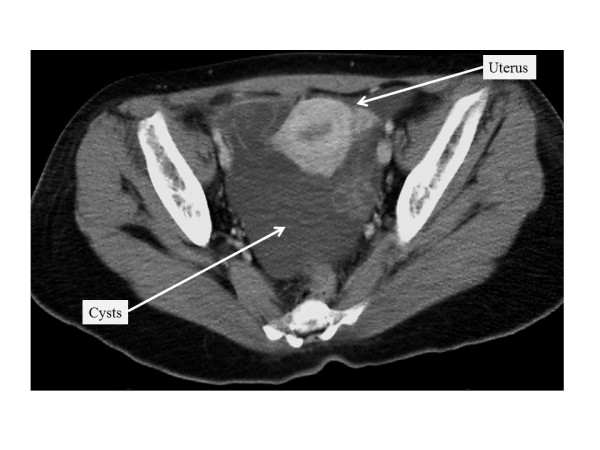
Computed tomography of the pelvis two years and nine months after the first surgery. There were multiple cysts in the pelvis.
